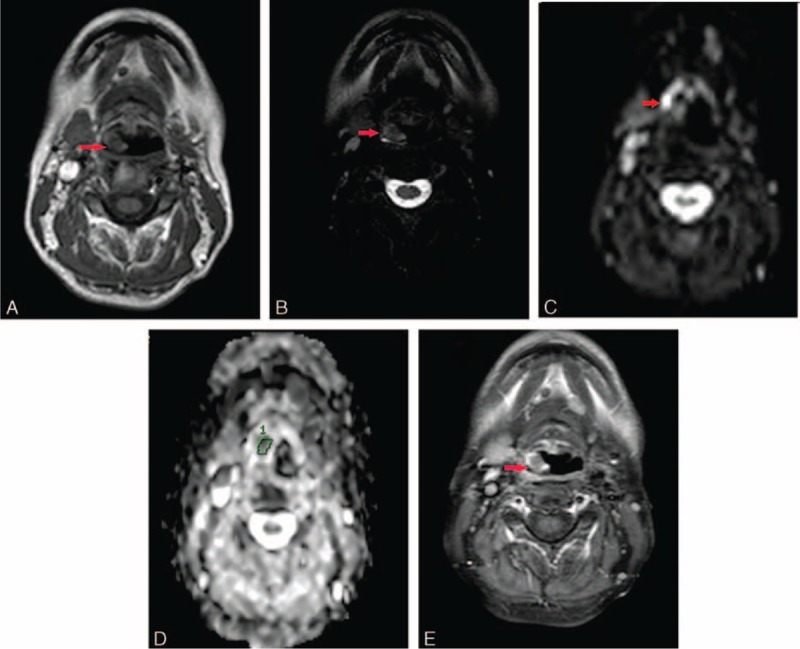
MRI revealed a 0.8 × 1.3-cm lesion in the epiglottis. T1-weighted imaging was isointense (A), but T2-weighted imaging was hyperintense (B). diffusion-weighted imaging exhibited high signal intensity (C). The apparant diffusion coefficient value was 0.741 × 10^−3^ mm^2^/s (D). The lesion exhibited heterogeneous enhancement (E). MRI = magnetic resonance imaging.
